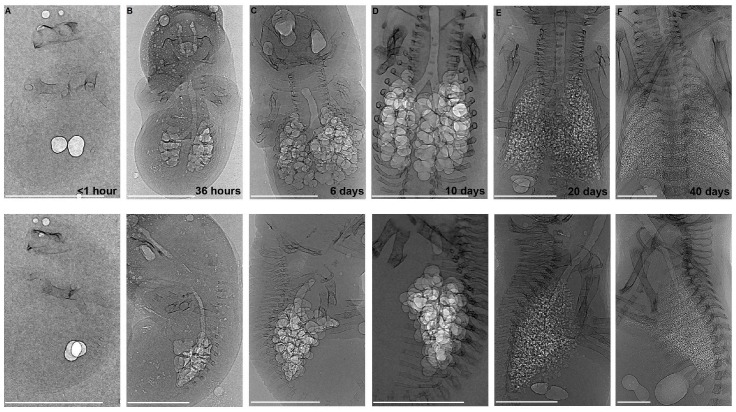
Phase contrast X-ray imaging of the developing fat-tailed dunnart using a synchrotron radiation source. Anterior-posterior (top) and lateral (bottom) views of the developing fat-tailed dunnart from birth (A) to 40 postpartum days (F). White areas indicate the less dense air-filled lung and trachea. Some air may also be noted in the nares (located at the top of the images) or on the body surface. The fat-tailed dunnart undergoes a substantial degree of extra-uterine lung development from just 2 ‘air bubbles’ visible after parturition (A). At P10 (D), the lungs are still characterised by large open circular sacs. By P20 (E) the size, shape and number of air sacs have substantially altered. Scale bar represents 1 mm.
